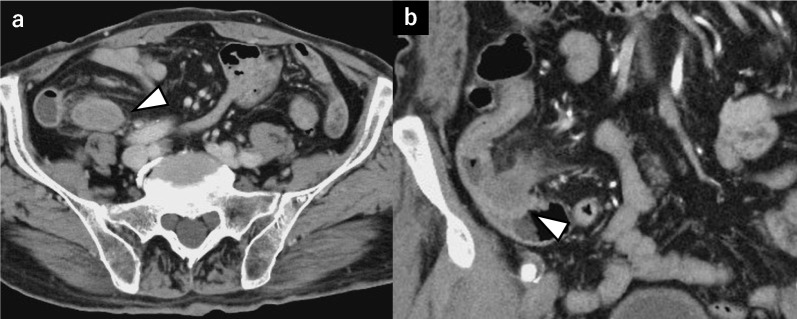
Contrast-enhanced computed tomography findings. a Axial image. A 27-mm mass is detected in the appendix. b Coronal image. The tip of the appendix is in contact with the small intestine, raising the possibility of tumor invasion
